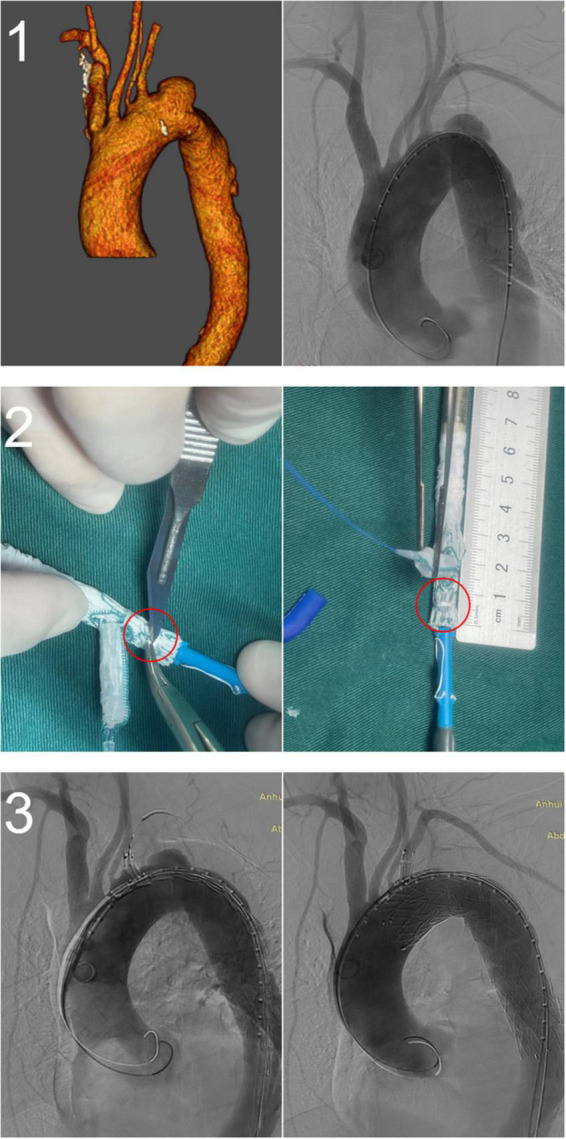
A fenestrated-branched stent graft was used to reconstruct the left subclavian artery (LSA) and the left vertebral artery (LVA). (1) Preoperative computed tomography angiography showing that the LVA originated from the aortic arch. (2) *In vitro* fenestration of the Castor stent graft. (3) Intraoperative and postoperative angiography showing the successful reconstruction of the LSA and LVA.
